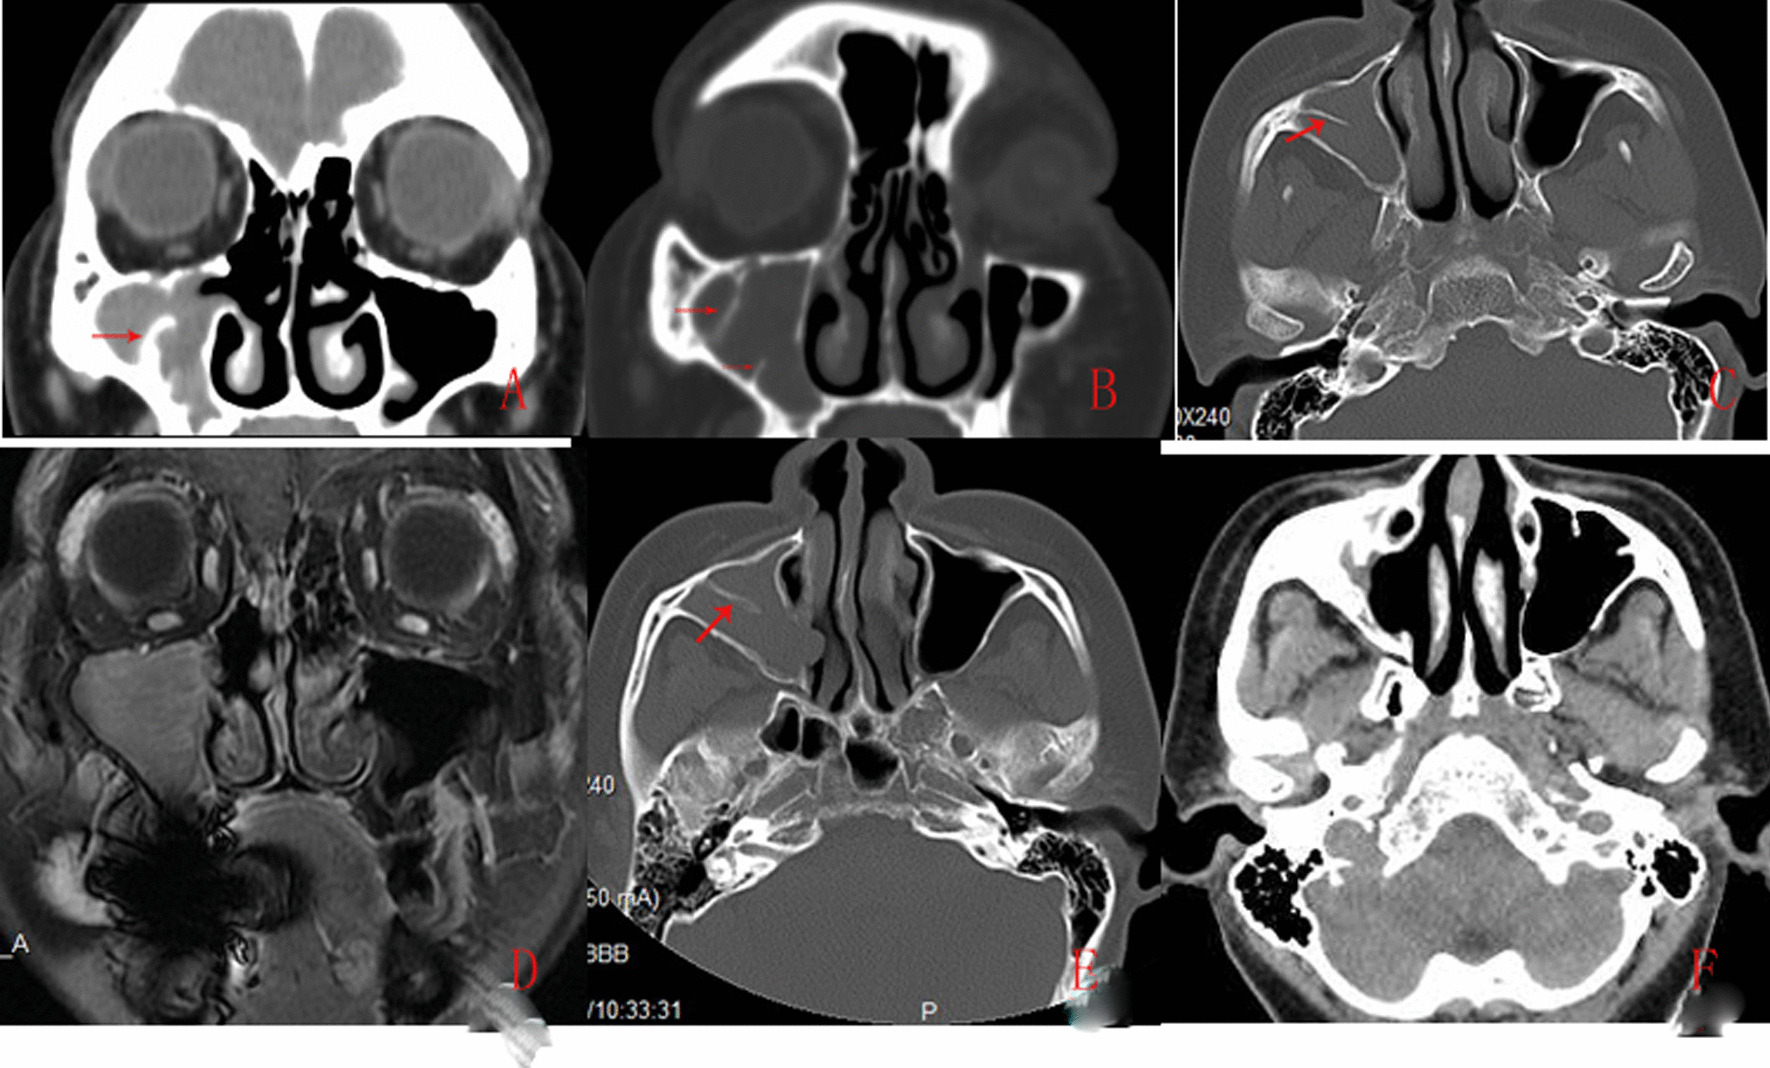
39 years-female. The first preoperative CT revealed hyperostosis and soft tissue shade of MS (A), the second postoperative CT revealed hyperostosis and IP recurrence at first postoperative 6 months (B), the second postoperative CT and MRI revealed hyperostosis and IP recurrence of MS (C and D) at second postoperative 6 months, the third preoperative CT revealed hyperostosis and IP recurrence of MS at second postoperative 10 months(E), the third postoperative CT revealed thickened mucosa at third postoperative 38 months (F). Red arrows indicated hyperostosis
